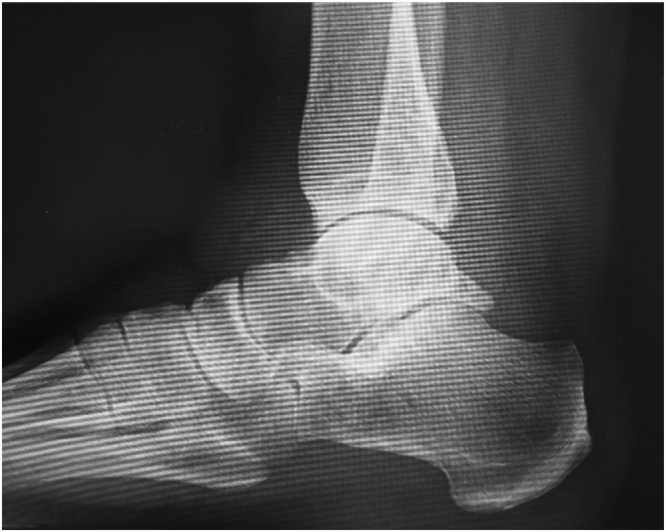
Lateral plain radiographic view of the left ankle obtained preoperatively showing the enlarged posterior talar process in the posterior ankle.
